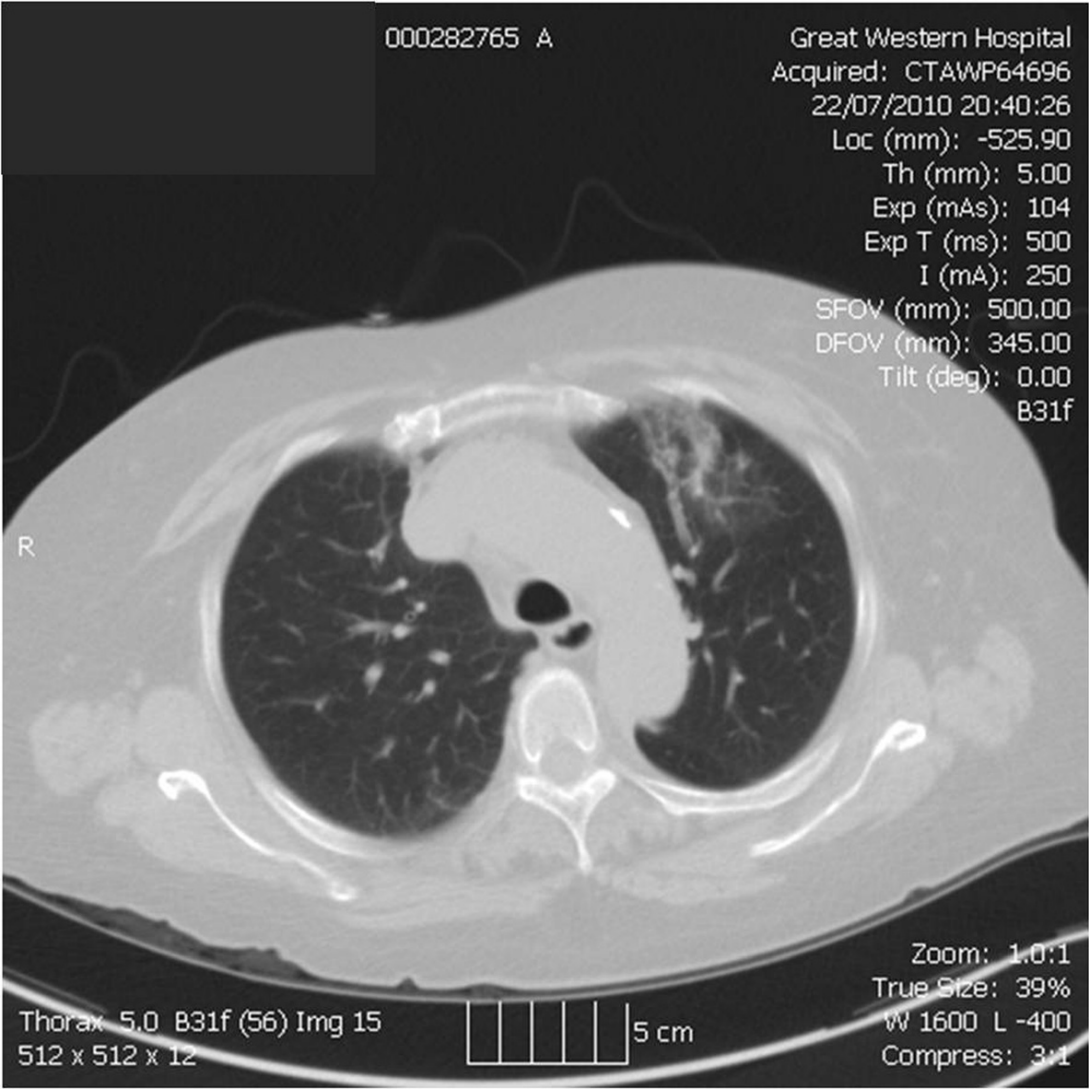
Computed tomography showing non-specific parenchymal distortion in the upper lobe of the left lung.
